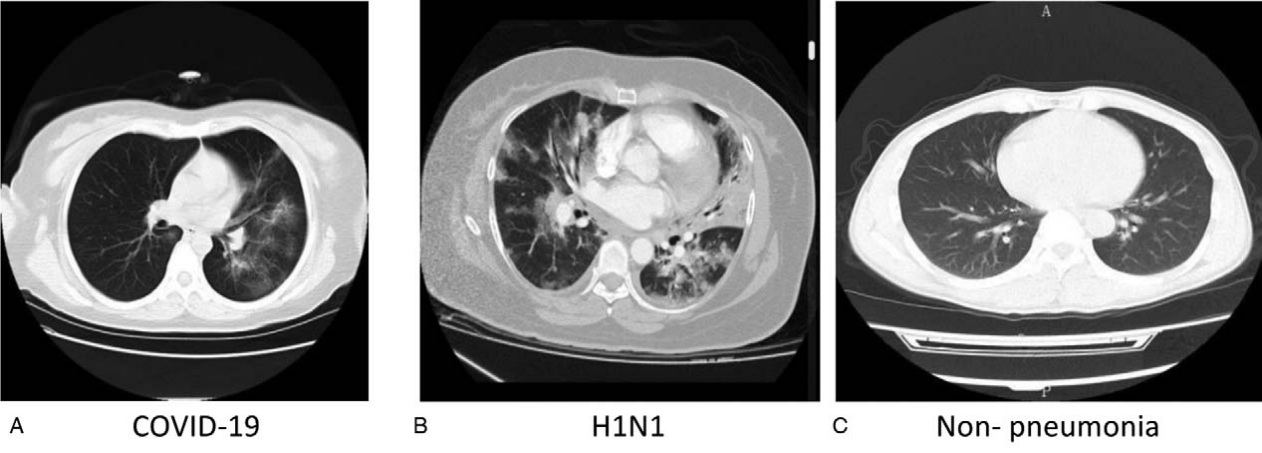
Typical cross-sectional CT image.
